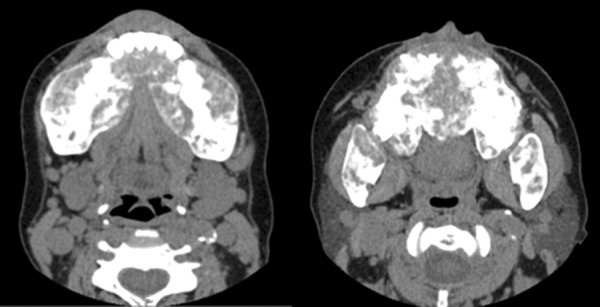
CT scan of the facial bones. The facial bones were enlarged, heterogeneous, sclerotic, and with some lytic areas, as well as bony expansion and tumefaction affecting most prominently the mandibular (left image) and maxillary areas, extending into the hard palate (right image).
